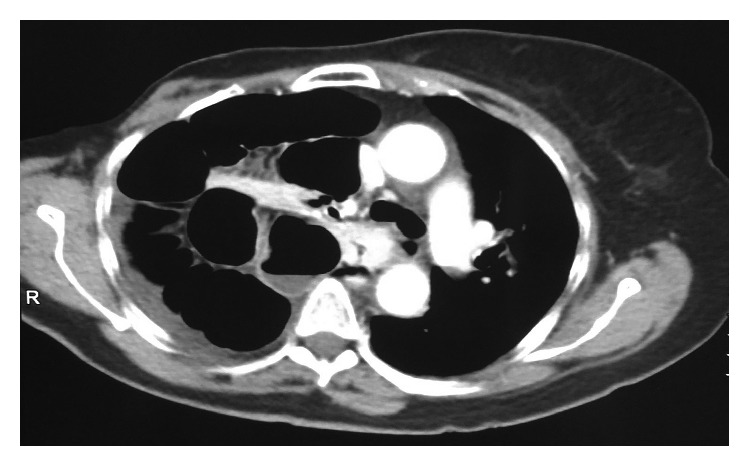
Abdomen and thorax CT scan. The findings confirmed the diagnosis of diaphragm traumatic herniation and the development of acute respiratory distress syndrome due to functional right pneumonectomy. Note that the right hemidiaphragm is absent and a large part of the small intestine is transported to the right side of the thoracic cavity.
